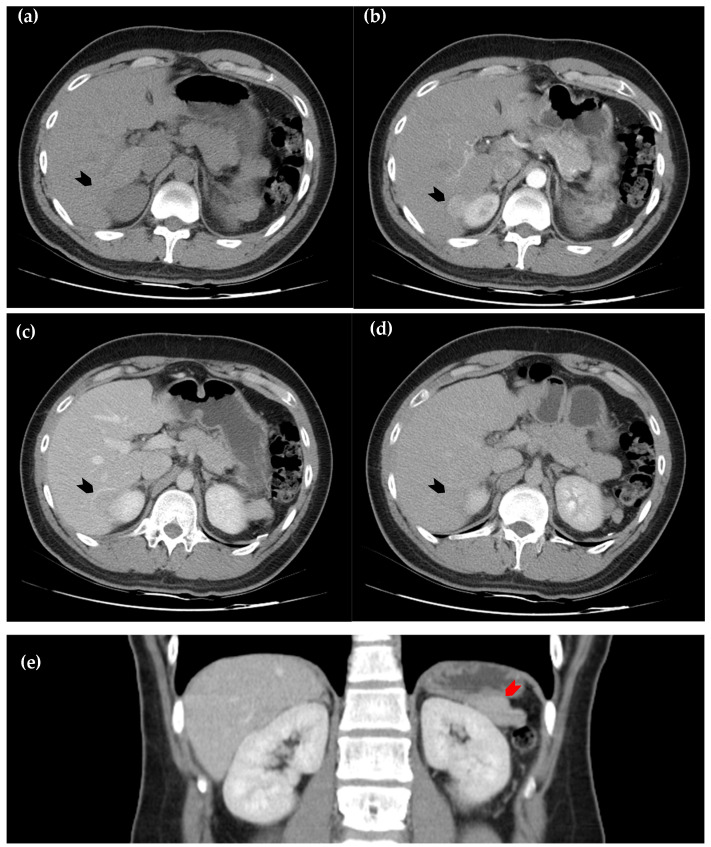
Dynamic computed tomography of the liver. (a) Axial view of the pre-contrast sequence shows a slightly hypodense lesion, as compared with the surrounding normal hepatic parenchyma, located in segment six of the liver (black arrowhead); (b) axial view of the arterial phase. The lesion exhibits homogeneous enhancement (black arrowhead); (c) axial view of the portal phase, in which the lesion becomes relatively hypoattenuating (black arrowhead); (d) the axial view of the delayed phase, in which hypoattenuation of the lesion persists but is less prominent than in the portal phase (black arrowhead); (e) coronal view of the portal phase, in which a soft tissue lesion at left perinephric area (red arrowhead) demonstrates similar attenuation to the intrahepatic lesion, raising suspicion for splenosis. However, due to the early wash-in and early wash-out enhancement pattern, hepatic tumors such as hepatocellular carcinoma and metastatic lesions remain important differential diagnoses [1,2,3,4].
